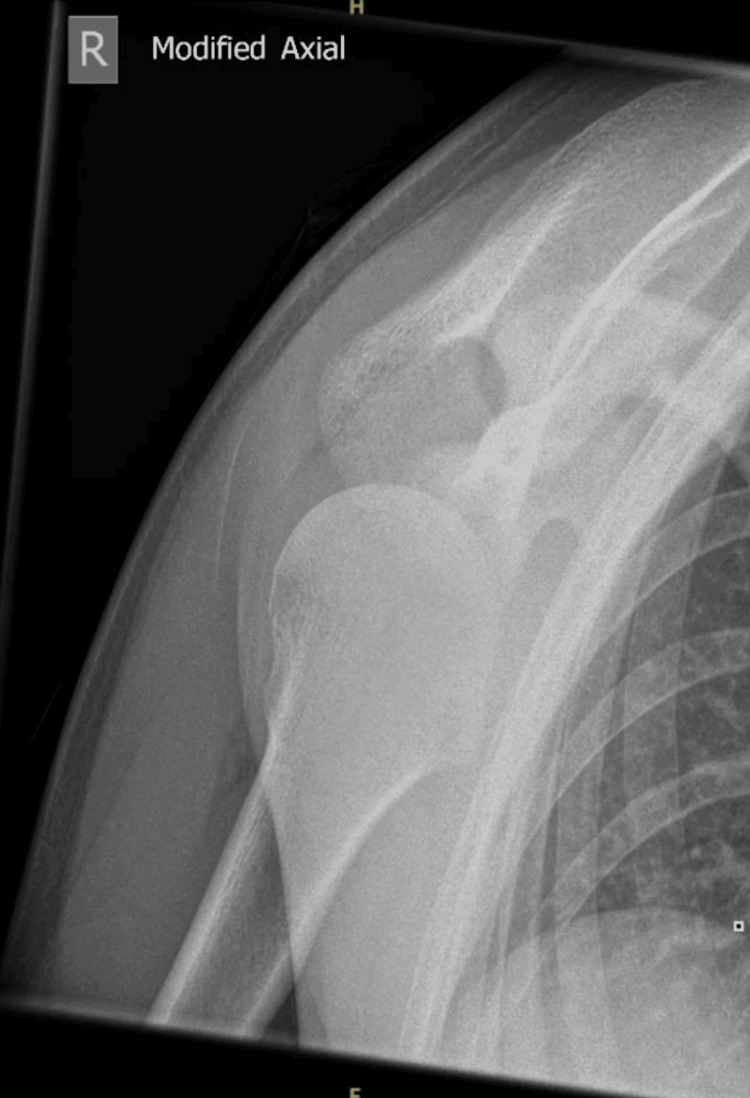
Lateral shoulder radiograph showing AC joint displacement with an associated coracoid fracture fragment AC: acromioclavicular
